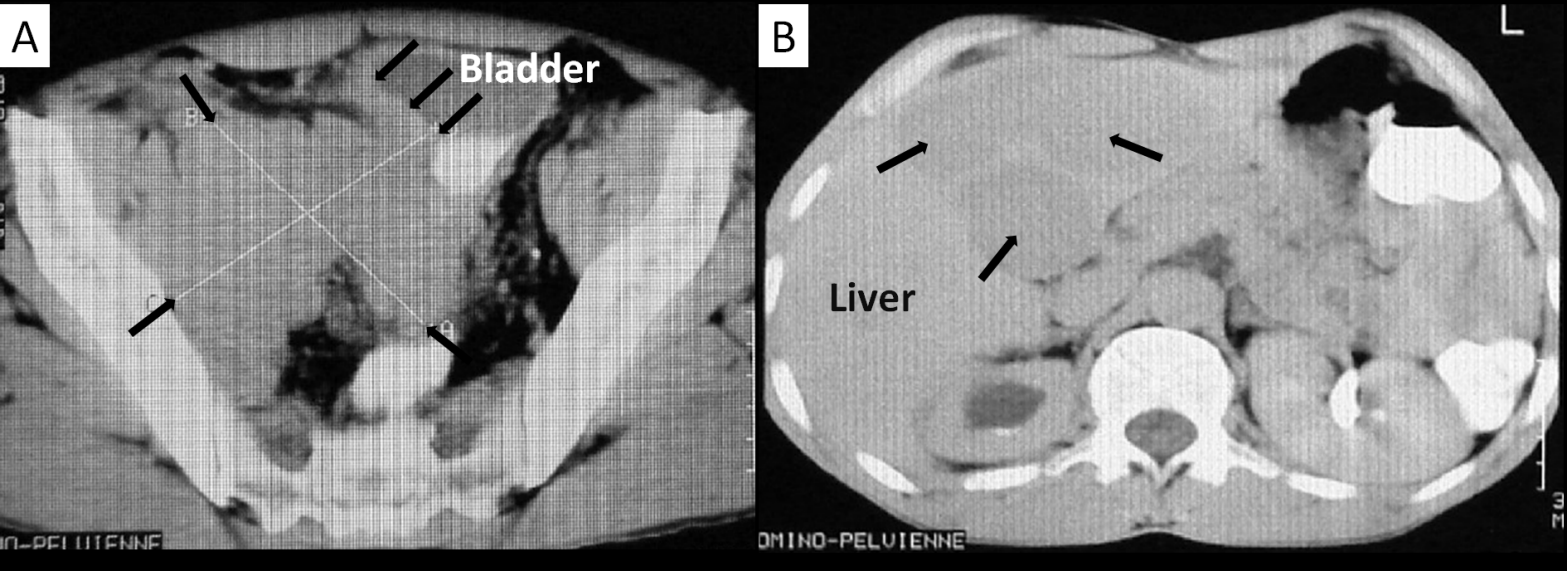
Bladder small cell carcinoma imaging [31]. A. Computed tomography scan of the pelvis shows a heavy tumor at the right bladder wall with intraluminal and extravesical extension (arrows). B. Computed tomography scan of the abdomen shows a multinodular liver disease from bladder small cell carcinoma (arrows).
